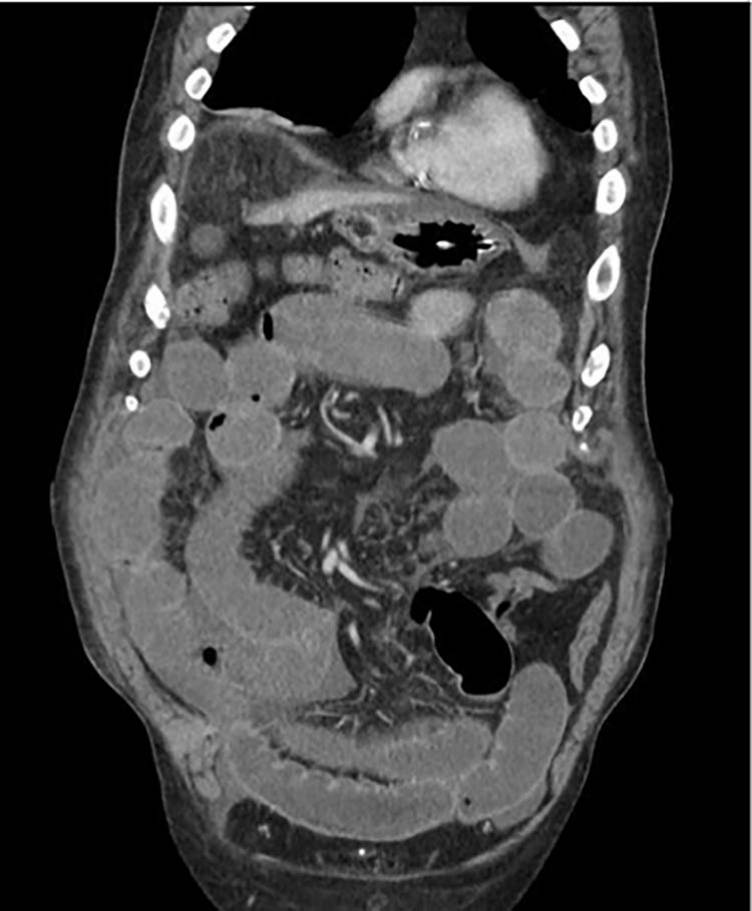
Repeat CT scan after attempted conservative management.
